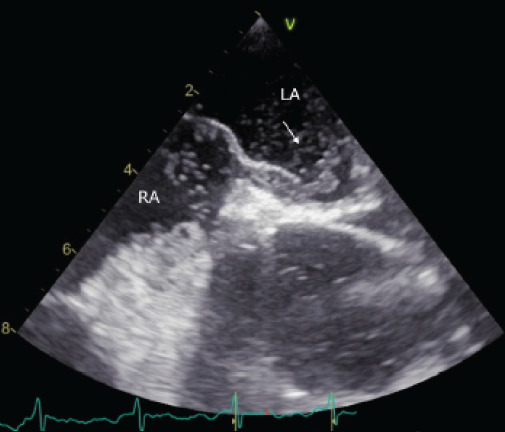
Transesophageal echocardiography, mid-esophageal bicaval view with agitated saline contrast. Microbubbles are visualized crossing from the right atrium (RA) to the left atrium (LA), confirming a large right-to-left shunt. A white arrow highlights the bubbles entering the left atrium.
